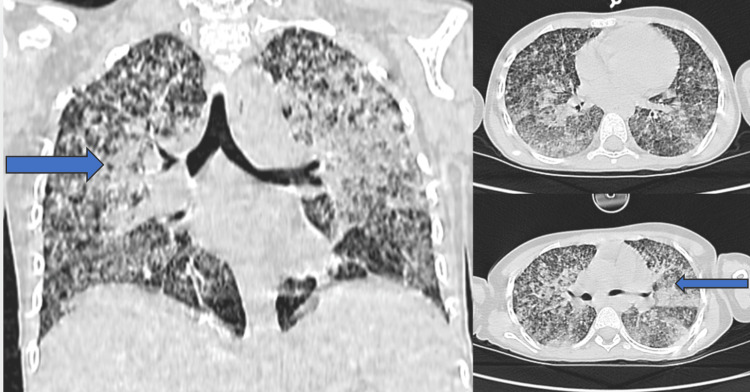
Chest CT scan showing a miliary tuberculosis pattern (left: coronal view; right: two axial views)
